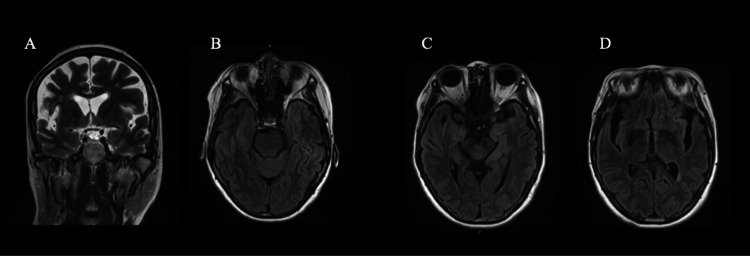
Magnetic resonance images of the patient’s brain. (A) Coronal view (T2-weighted image): mild atrophic changes are observed in both cerebral hemispheres. (B)–(D) Axial views (FLAIR: fluid-attenuated inversion recovery): No apparent abnormal signal was observed in (B) cerebellar vermis, (C) cerebral aqueduct, or (D) medial thalamus and periventricular region of the third ventricle.
